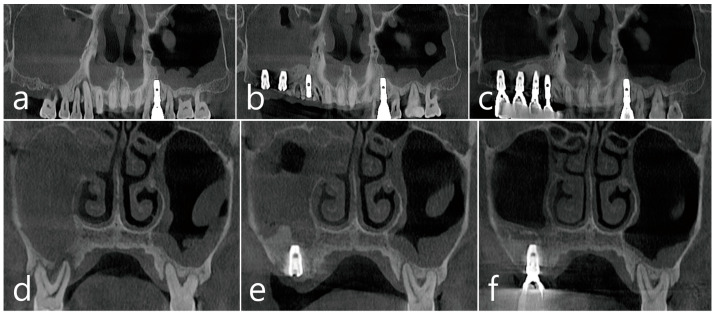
Case 2. Panoramic CBCT images before surgery (a), immediately after surgery (b), and 1 year after the prosthetic loading (c). Coronal CBCT images before surgery (d), immediately after surgery (e), and 1 year after prosthetic loading (f). Sinus opacification and nasal polyp disappeared in the images obtained 1 year after the loading. CBCT; cone beam computed tomography.
